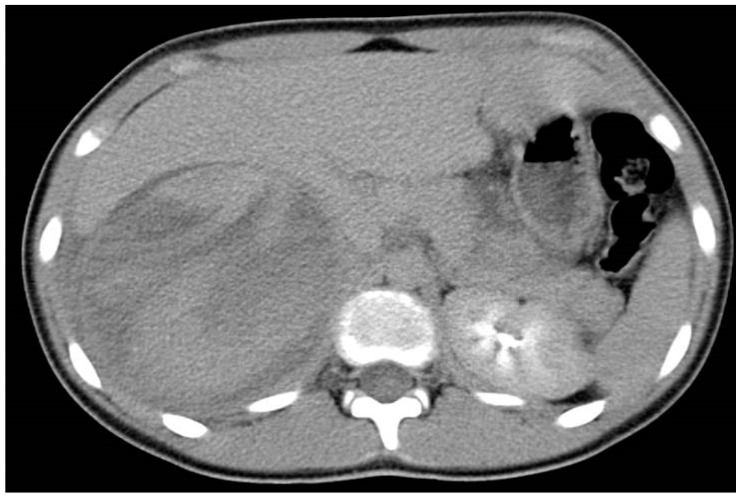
CT with intravenous contrast shows a laceration of the right kidney with perirenal hematoma.
